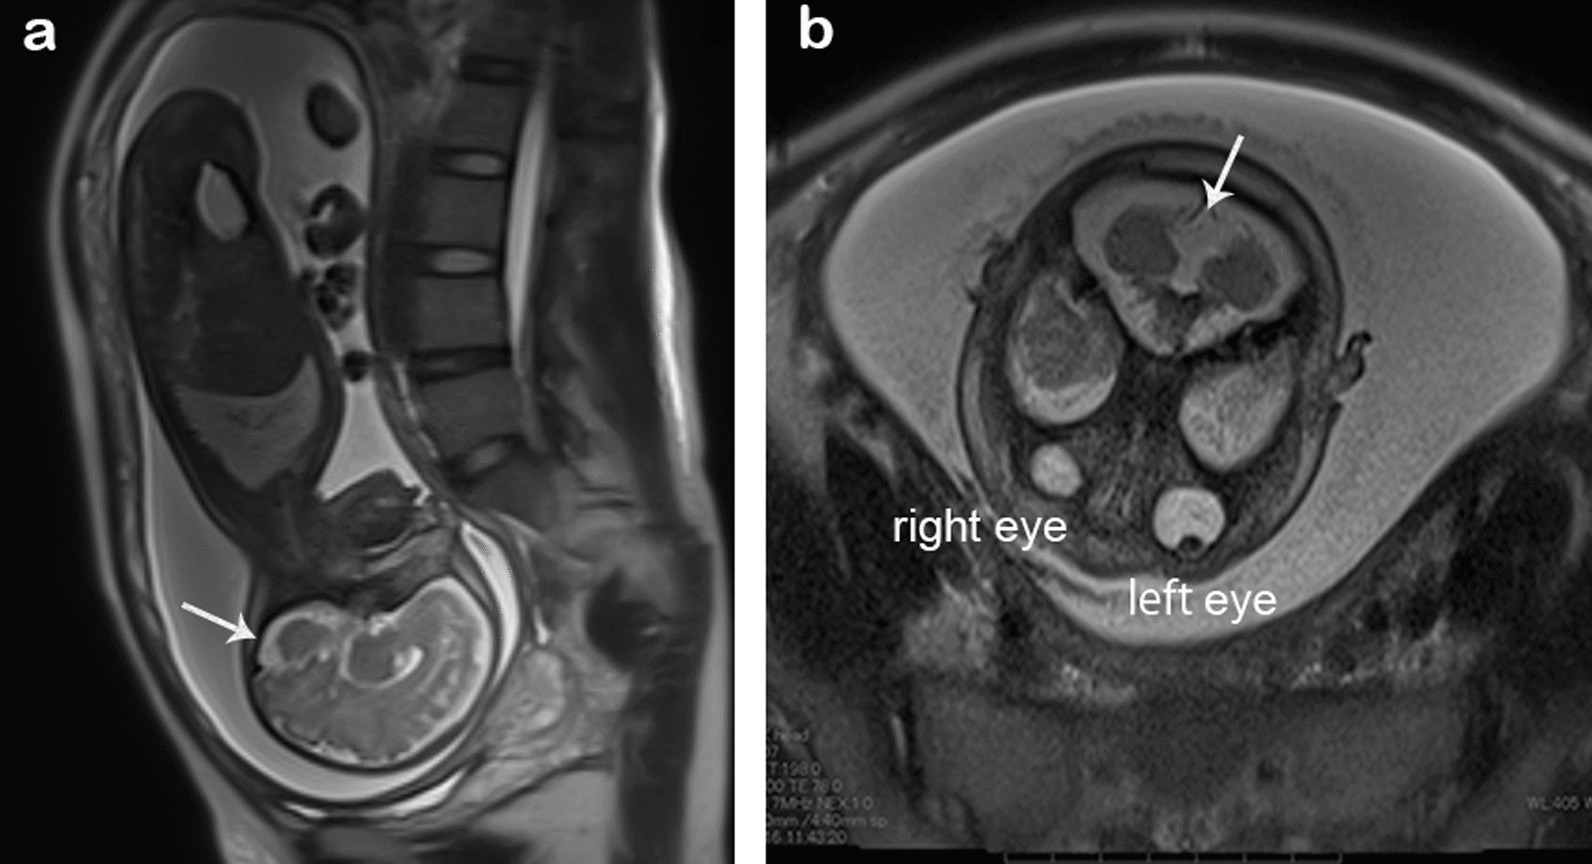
Fetal magnetic resonance imaging scans. Sagittal (a) and axial (b) views at 34 weeks of gestation. Arrows indicate the cerebellum
